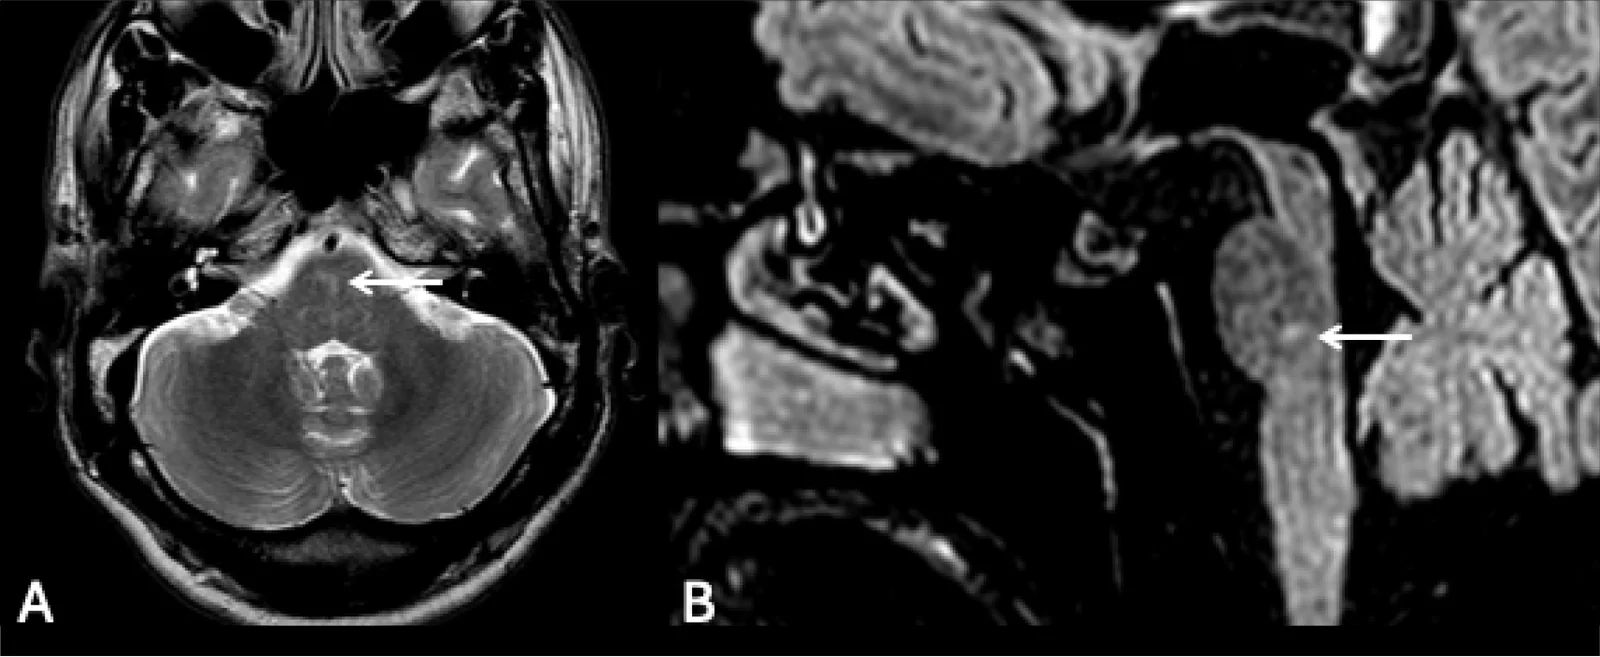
MRI of a 27-year-old man presenting with cognitive-behavioral
